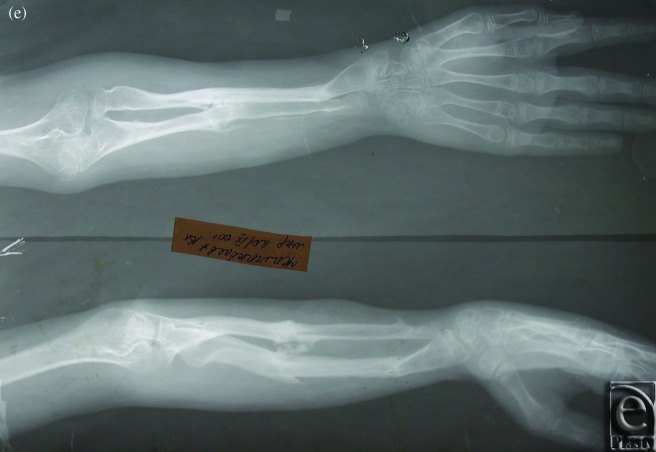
Case 1: Congenital pseudarthrosis forearm. (*a*) Preoperative view, (*b*) Preoperative x-ray, (*c*) External fixation, (*d*) The 1-year postoperative view, and (e) A 1-year postoperative x-ray.
